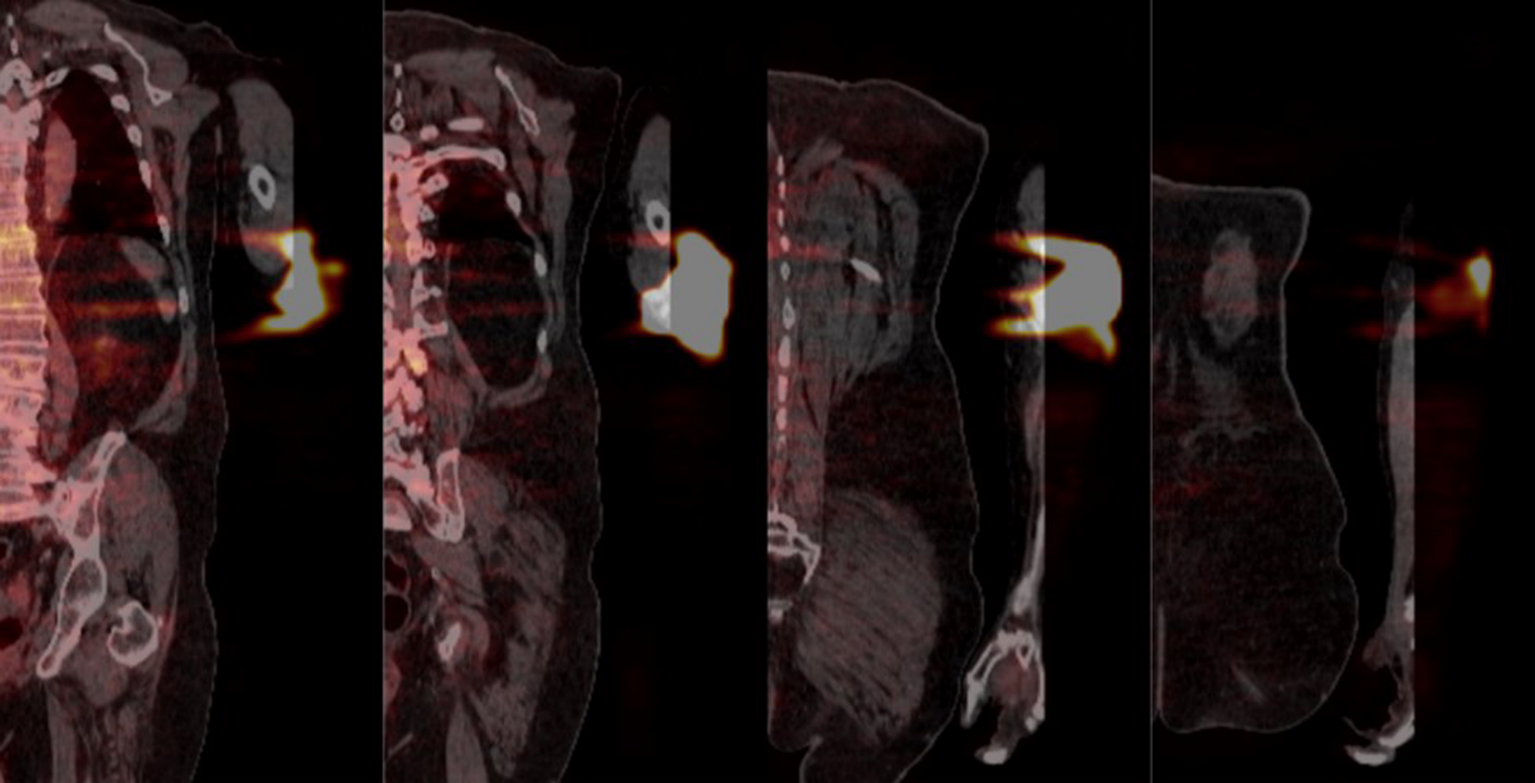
Coronal PET/CT views from anterior to posterior (left to right respectively) detailing the extravasated area. The distance between successive slices shown is approximately 40 mm.
